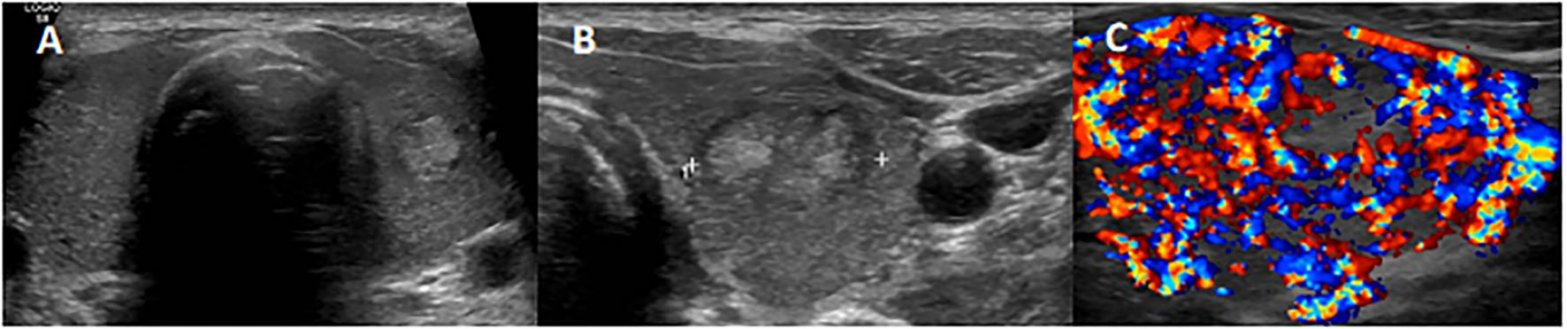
Thyroid ultrasonography (US) examination showing a non-uniform hypoechoic glandular parenchyma (A), with a 17-mm well-defined hyperechoic nodule in the left lobe (B) and a diffused markedly accentuated vascularization as described in thyroid storm (C).
